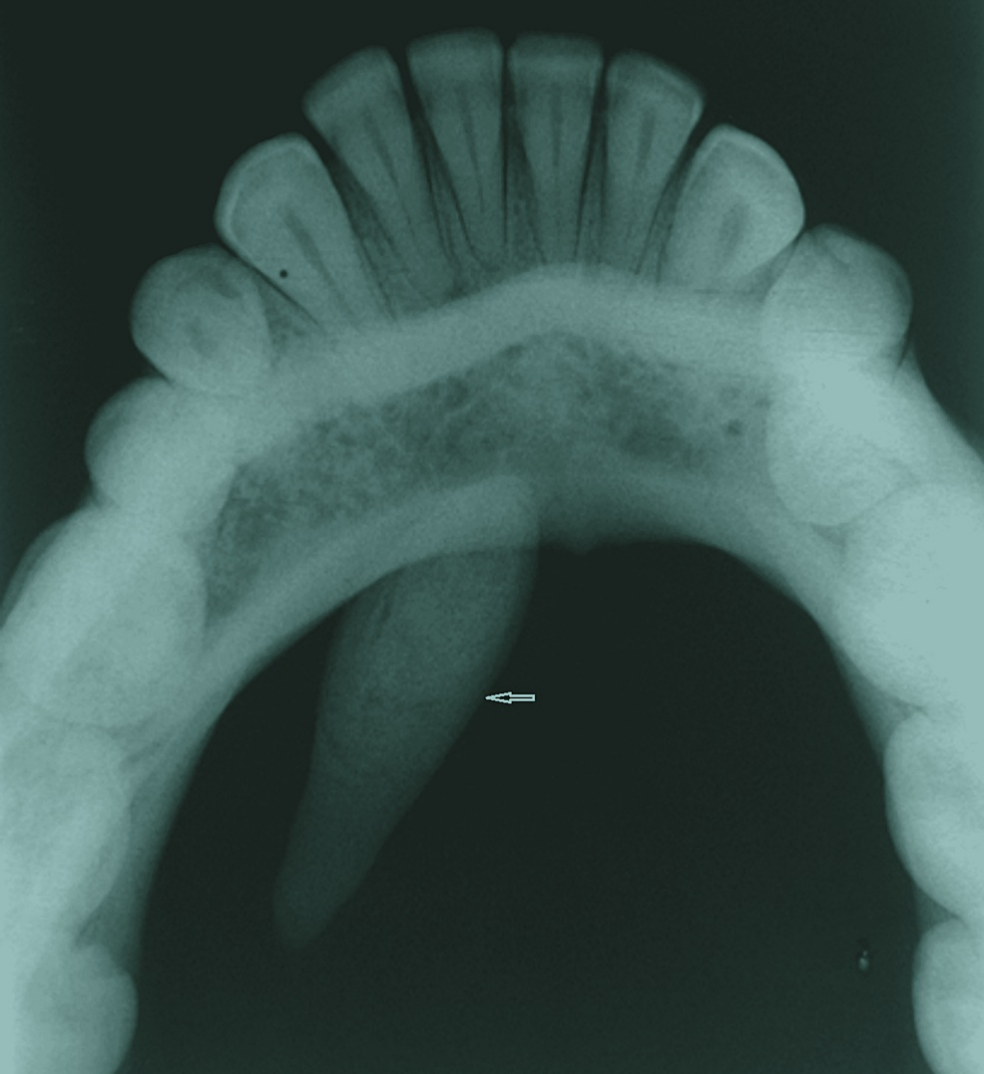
Topographic mandibular occlusal radiograph Topographic mandibular occlusal radiograph showing an unusually large sialolith (white arrow) on the right side of the floor of the mouth.
